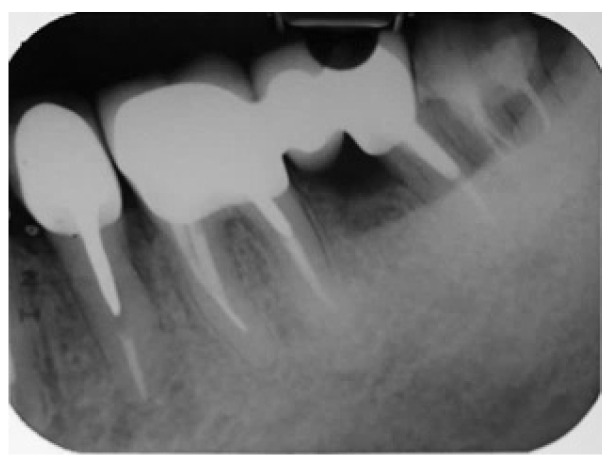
Five years follow-up periapical X-ray. Stable crestal bone level and radiographic bone fill in the previously extracted area.
